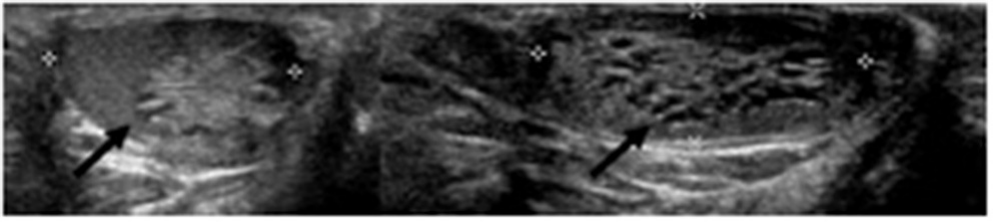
Pre-operative ultrasound of present case. Cysts in the right mediastinum testis (black arrow).
